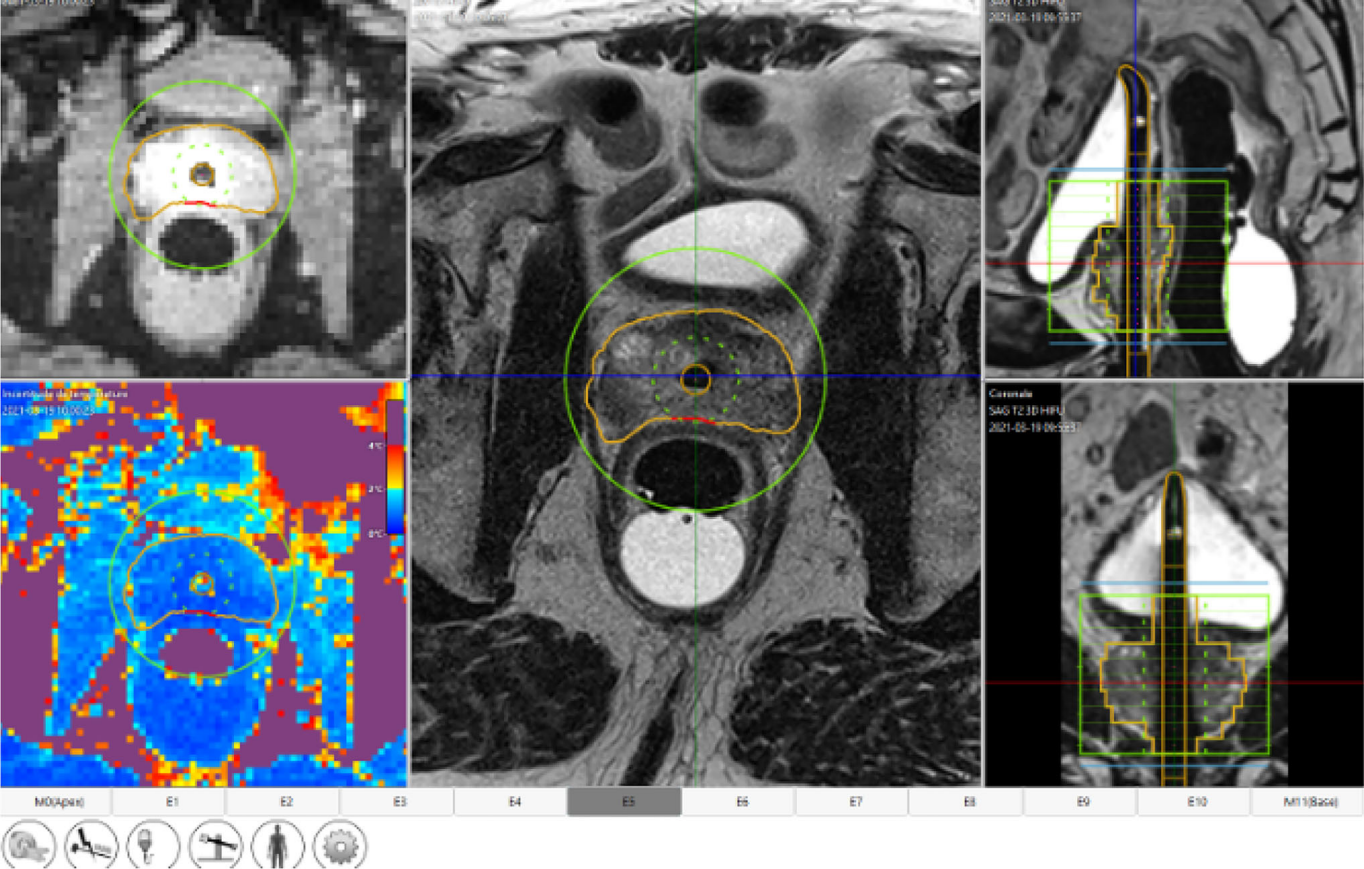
Treatment planning. The radiologist can draw the desired treatment boundary (orange line) on the axial T2-weighed MR image for each transducer element separately. The transducer within 3 mm of urinary bladder neck and external urinary sphincter can be deactivated to prevent thermal injury. The quality of MR thermometry can also be evaluated at this stage. The colour on the MR thermometry quality map (left lower corner) represents change in temperature from baseline, with dark blue indicating 0 °C change, i.e. good quality, and dark red indicating 4 °C change, i.e. suboptimal quality
